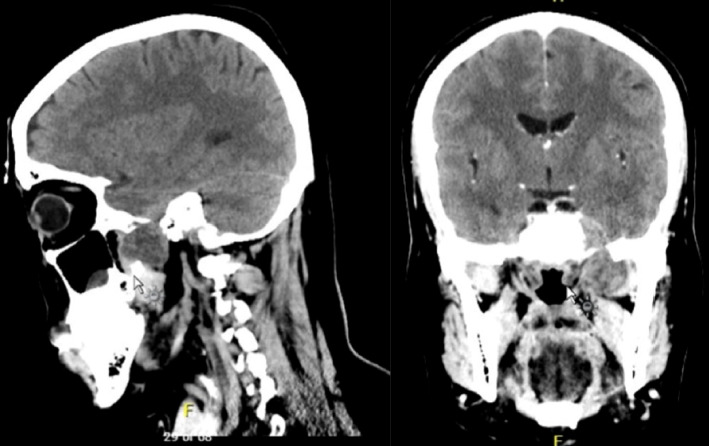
Sagittal and coronal CT views showing trigeminal schwannoma with intracranial extension.
